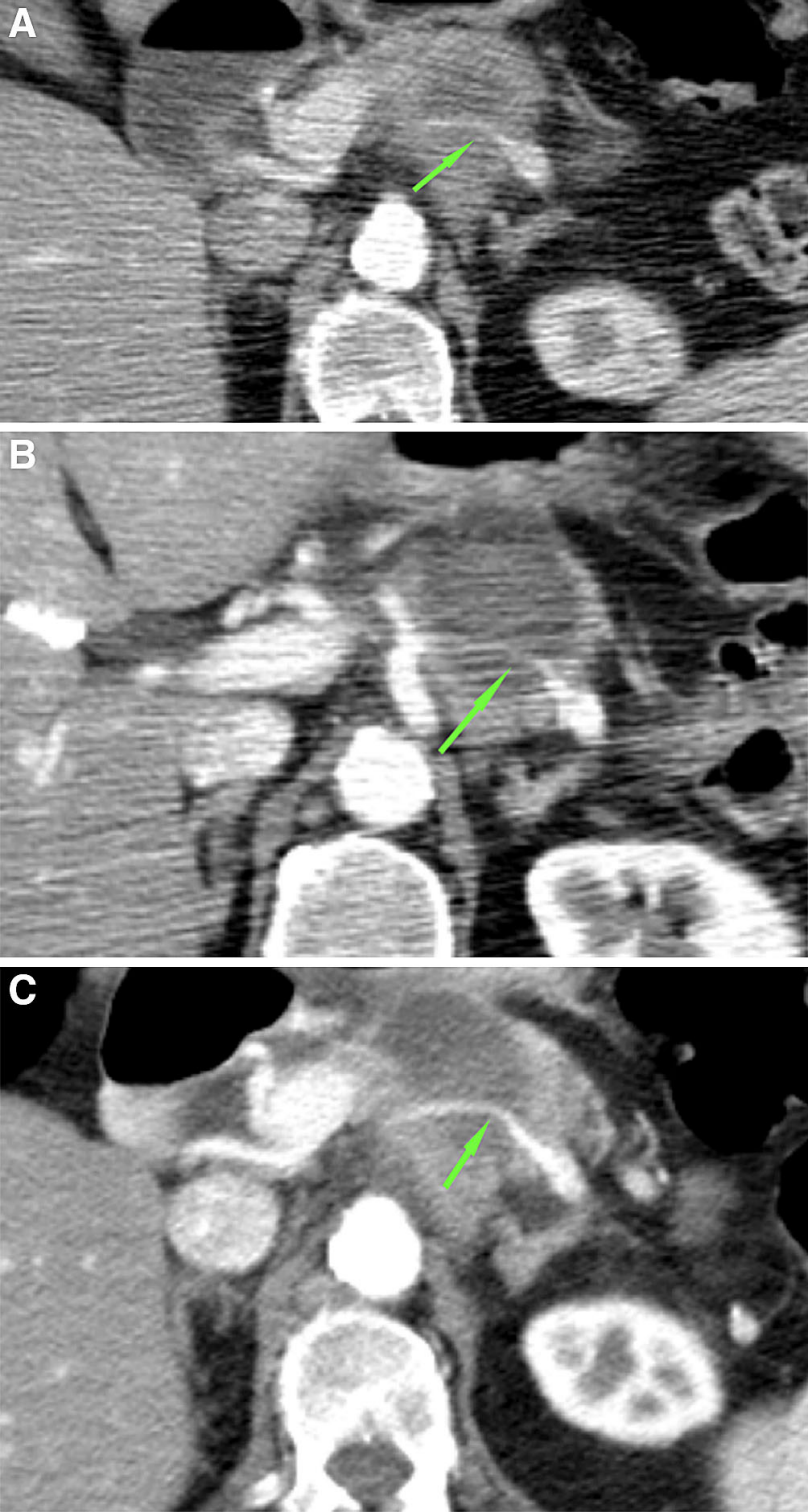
CT-images in the axial plane, of a 76-year-old woman with a ductal adenocarcinoma in the pancreatic corpus. A The splenic artery is encased by the tumor but still patent(arrow). B One week after RFA, the splenic artery is in contact with the ablation zone, but still patent (arrow). C This remained unchanged at follow-up 1 month later (arrow)
